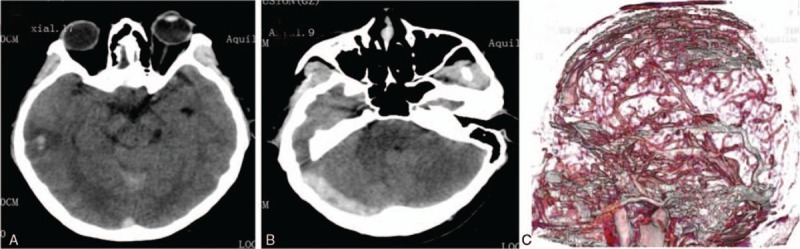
The results of 320CT before operation. A. Noncontrast CT head scan showed both subarachnoid and intraparenchymal hemorrhage and brain edema at the right temporal lobe; B. Noncontrast computed tomography head scan showed spontaneous hyperdensity of right transverse sinus; C. CT venography showed a contrast filling defect in the superior sagittal sinus and right transverse sinus. CT = computed tomography.
